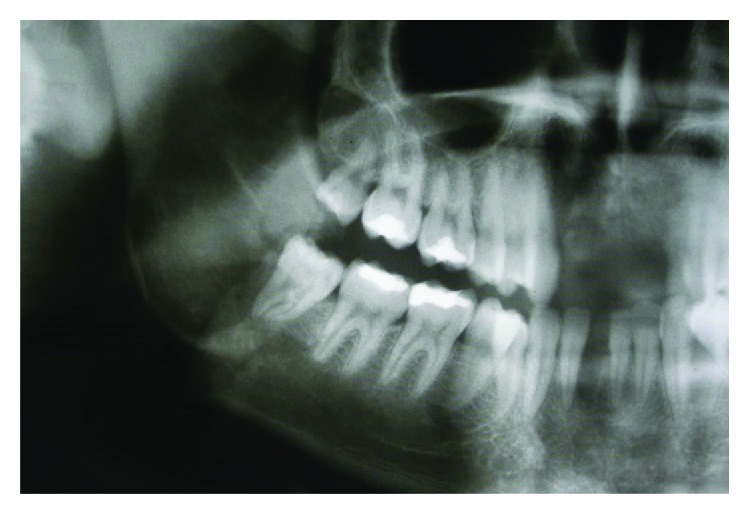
Orthopantomography demonstrating incorrect union of the fractured extremities in the right quadrant mandibular angle.
